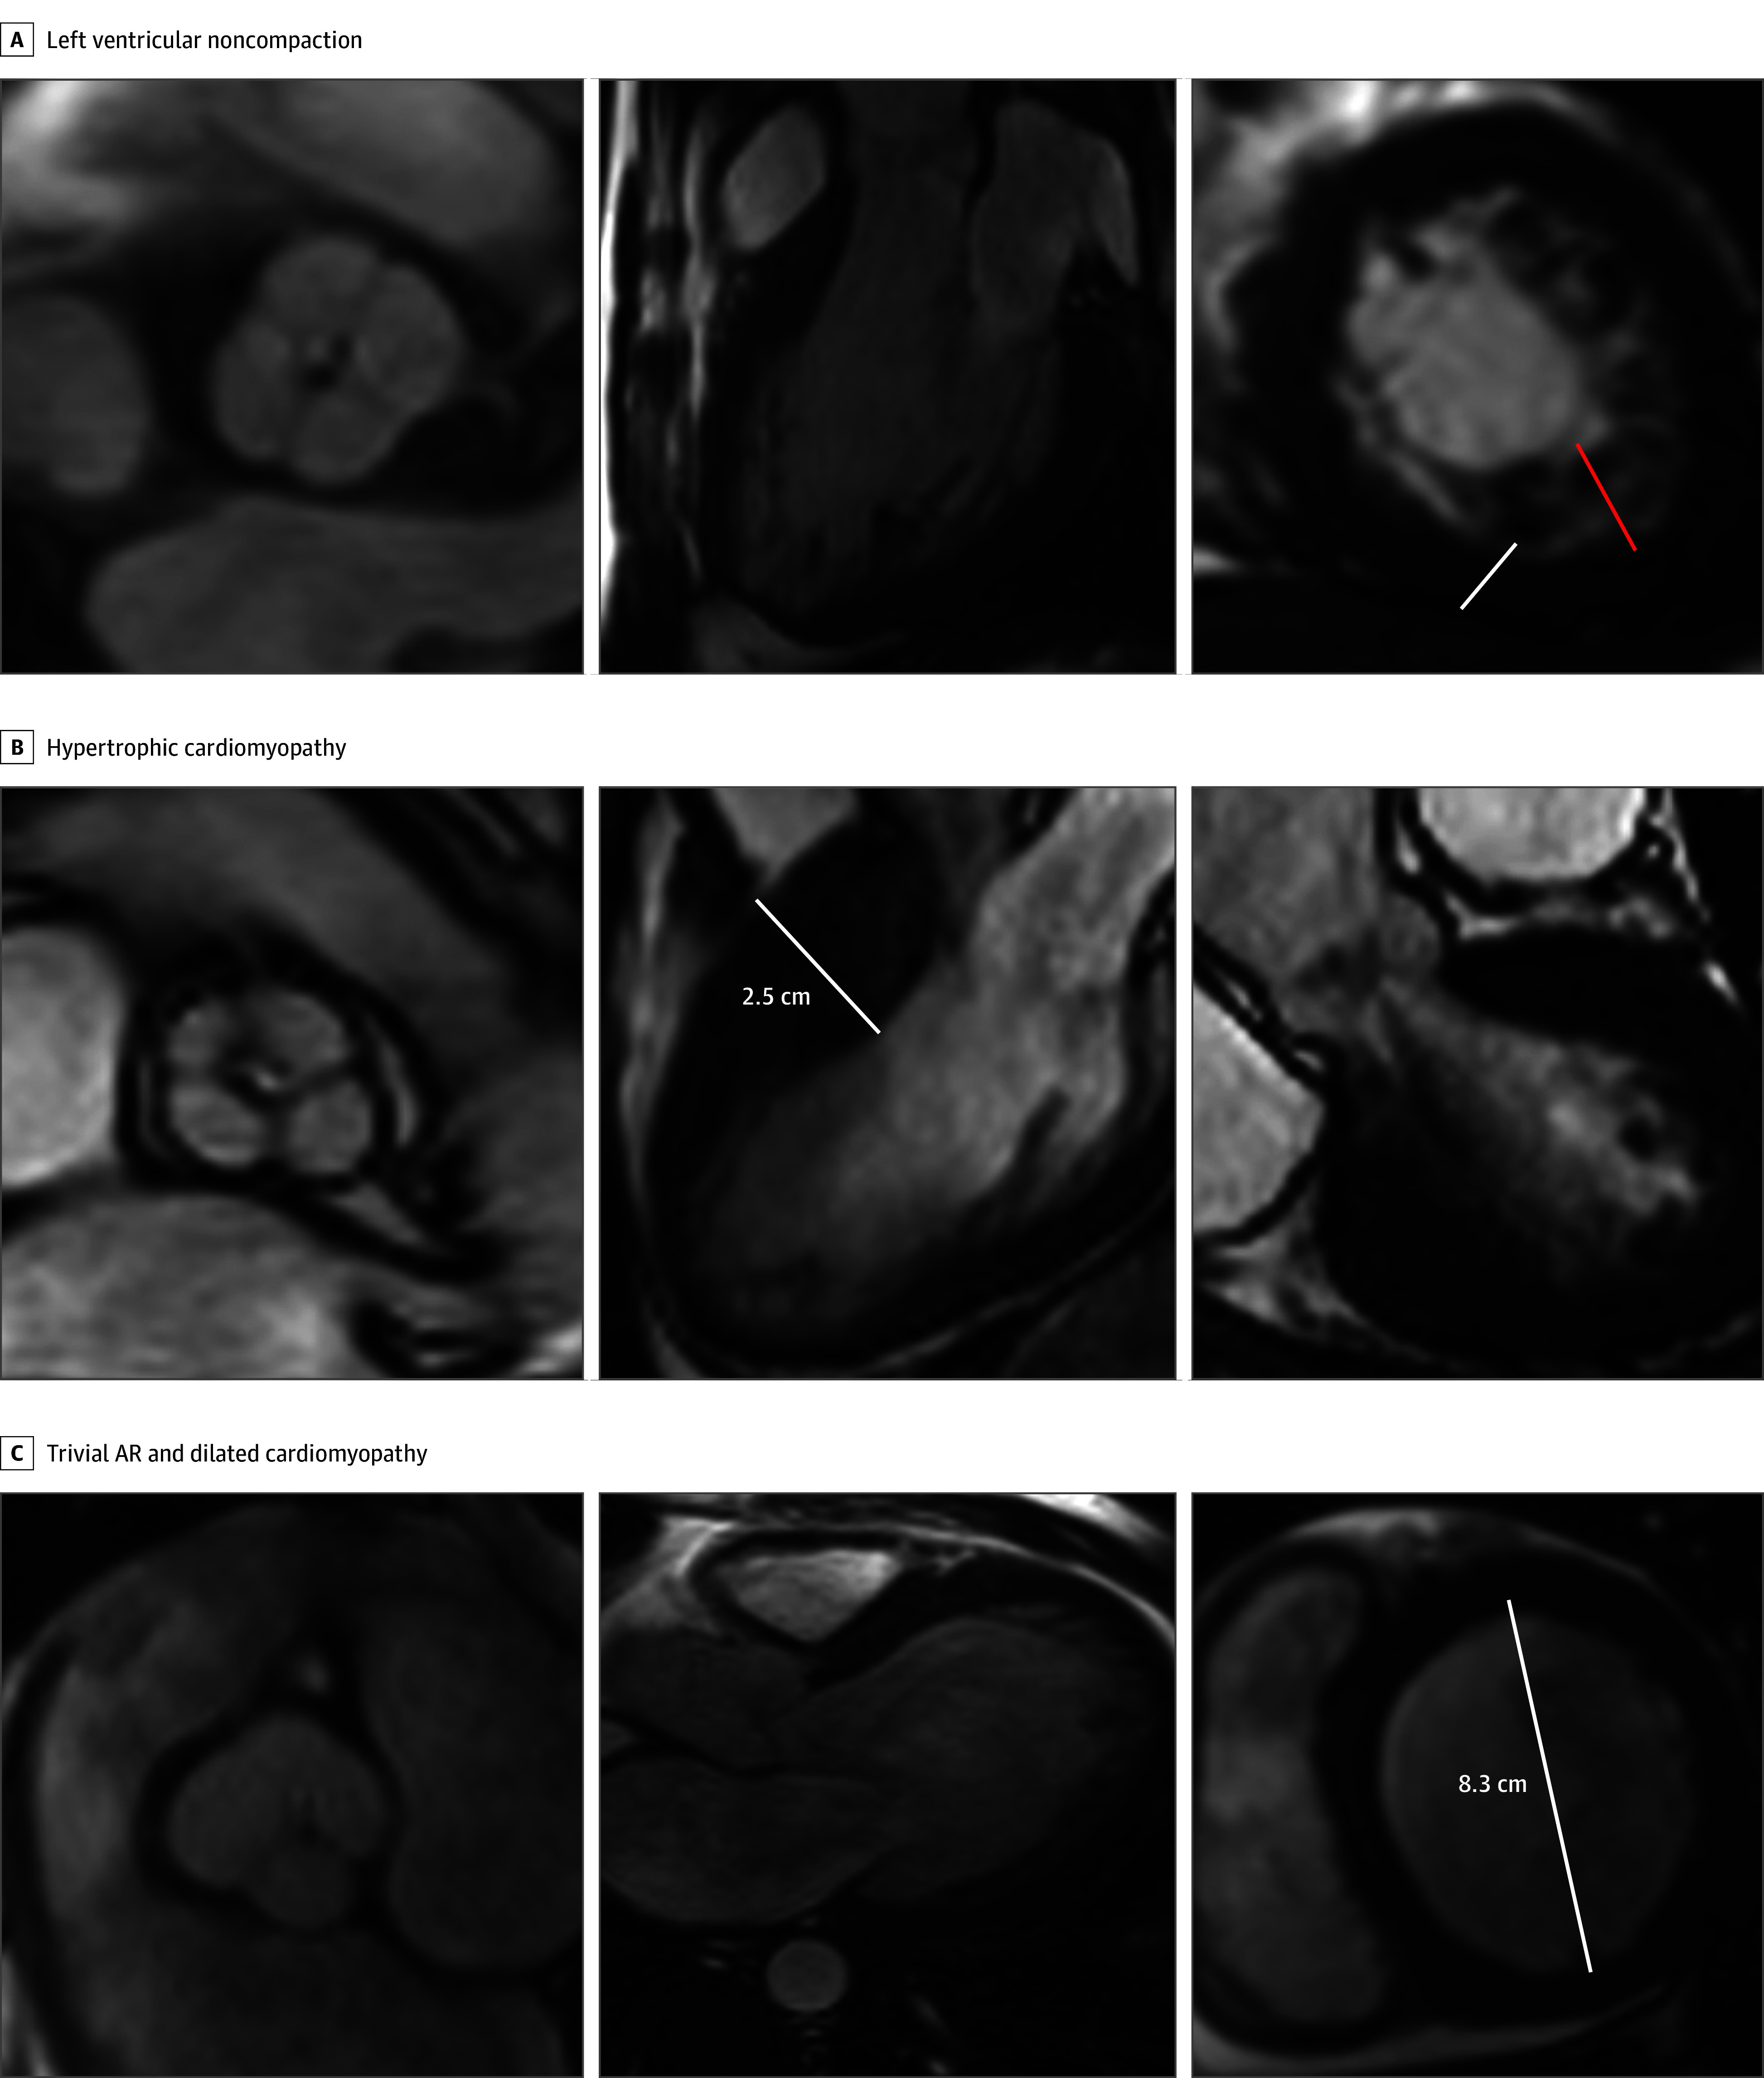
Coexistence of Quadricuspid Aortic Valve (QAV) and Cardiomyopathy Detected From Cardiac Magnetic Resonance Imaging A, Patient with QAV diagnosed with left ventricular (LV) noncompaction with a noncompacted (red line) to compacted (white line) ratio greater than 2. B, Patient with QAV diagnosed with hypertrophic cardiomyopathy with interventricular septal thickness of 2.5 cm (white line). C, Patient with QAV diagnosed with trivial aortic regurgitation (AR) and dilated cardiomyopathy with cavity size of 8.3 cm (white line). Images on the left depict the cross-sectional aortic valve view and the middle images, the LV 3-chamber view. Images on the right in A and C display the true LV short-axis view and in B, a coronal view of the LV outflow tract.
